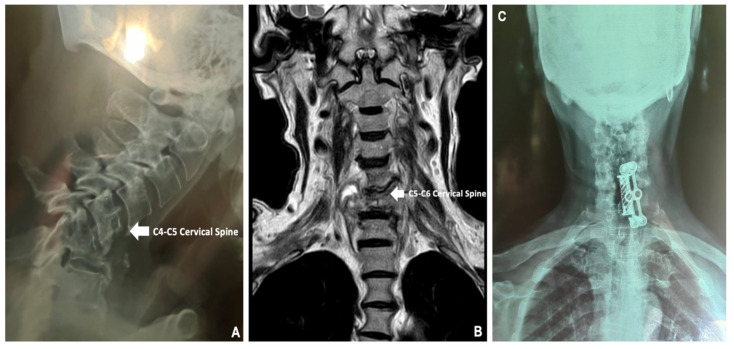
(A): Lateral X-ray of the cervical spine showing crushing of the C5 and C6 vertebral bodies. (B): Simple MRI up to T6, showing crushing of the C6 and C7 cervical bodies. (C): Anterior cervical spine fixation with threaded basket and automatic titanium locking.
